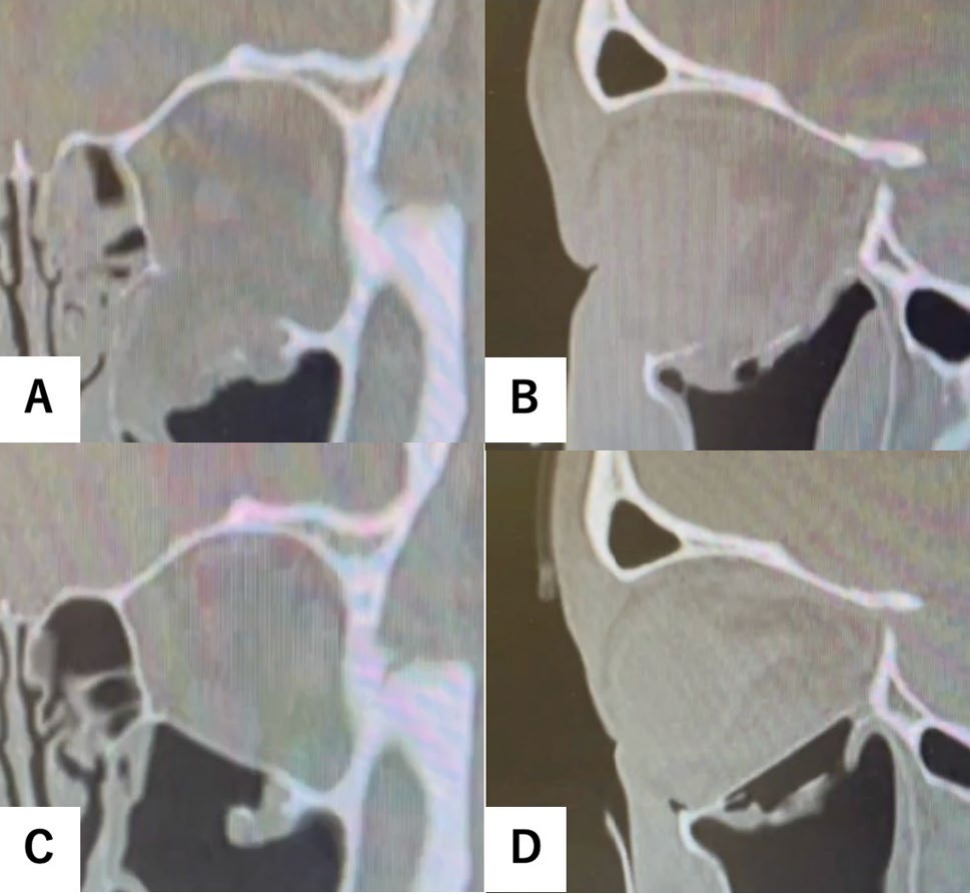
Computed tomography (CT) images before and after surgery. Images A and B are reformatted CT images before surgery. The slope of the posterior end of the orbital floor was fractured. Images C and D are reformatted CT images after surgery. The orbital contents were restored with complete reconstruction of the orbital floor, even with a fracture in the slope
